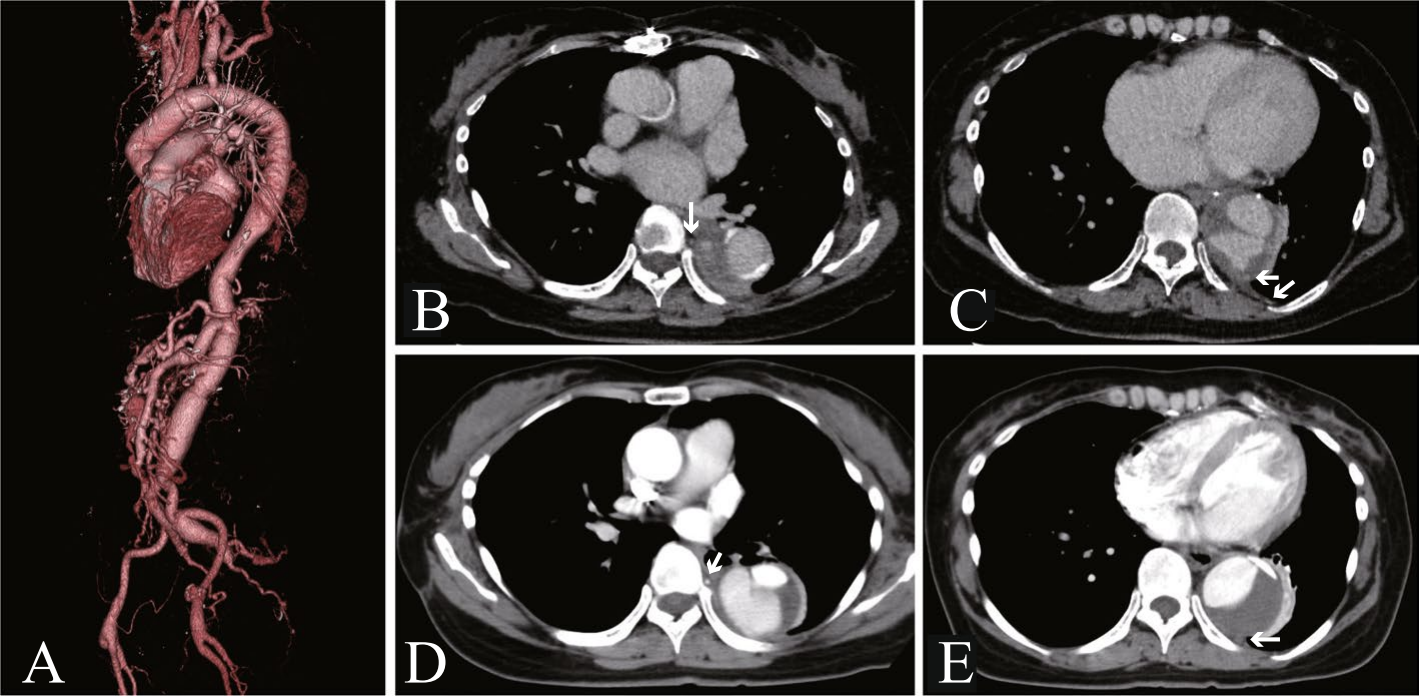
Computed tomography images of the aneurysm. A A 3D computed tomography (CT) image of the whole aorta. B and C CT images showing the recurrent aneurysm. Two contrast extravasation sites detected in the venous phase were distant from the anastomotic site and linked to the right eighth (B) and left tenth (C) intercostal arteries, indicated by white arrows. D and E CT images before thoracoabdominal aortic replacement revealing that all intercostal arteries originated from the false lumen; the right eighth intercostal artery was open (D), while the left tenth was occluded by a thrombus in the false lumen (E). The right eighth and left tenth intercostal arteries are indicated by white arrows
